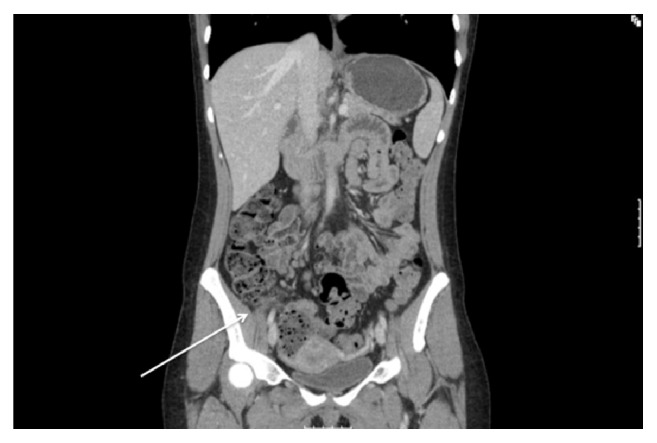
CT Scan of abdomen, supine view, demonstrating subtle loss of tissue plane between the caecal pole and surrounding areas.
